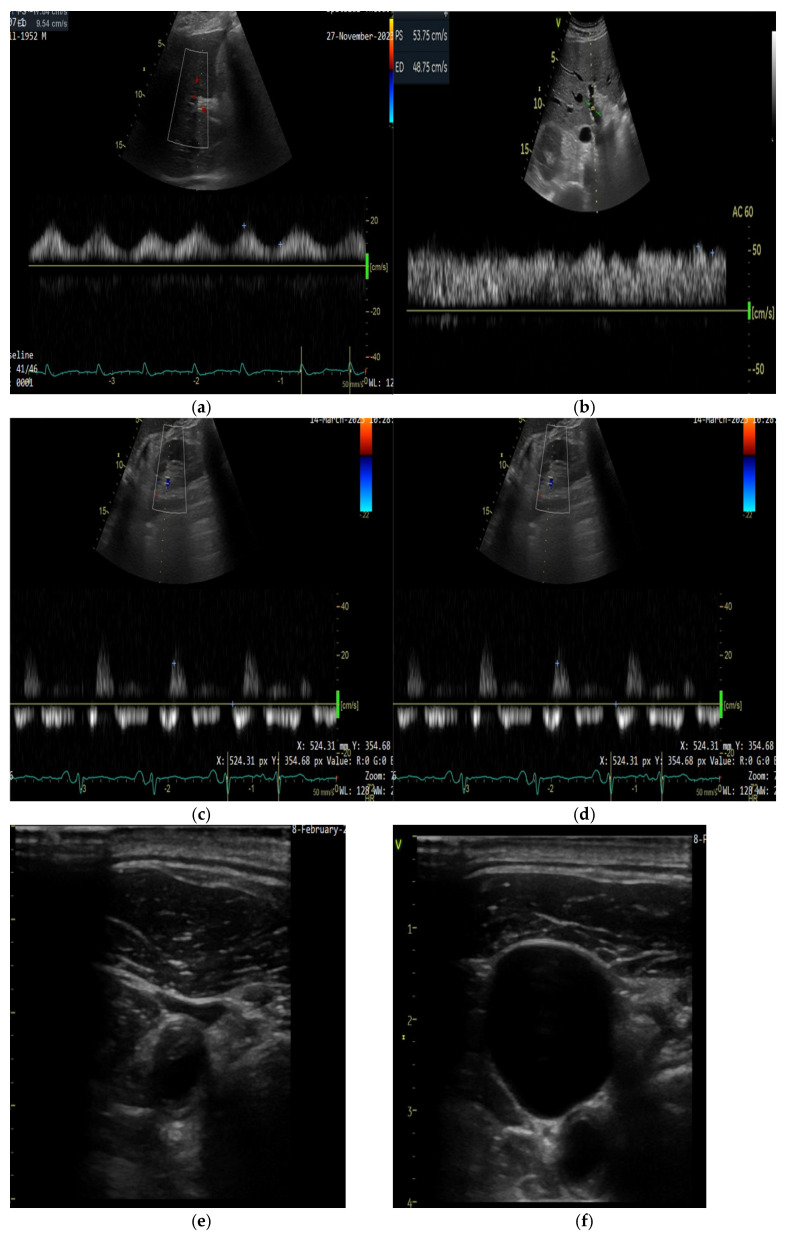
Sequential Doppler changes in different patients with ADHF. (a,b) Portal vein Doppler in patient with systemic congestion with pulsatile flow on admission (a), and normalized flow after decongestion (b). (c,d) Intra-renal venous Doppler in another patient with systemic congestion: discontinuous flow on admission (c), normalized flow to continuous pattern at discharge (d). (e,f) Jugular vein ultrasound in patient without systemic congestion: IJV diameter is small at rest (0.10 cm) (e) and increases up to 2.5 cm during Valsalva maneuver (f).
